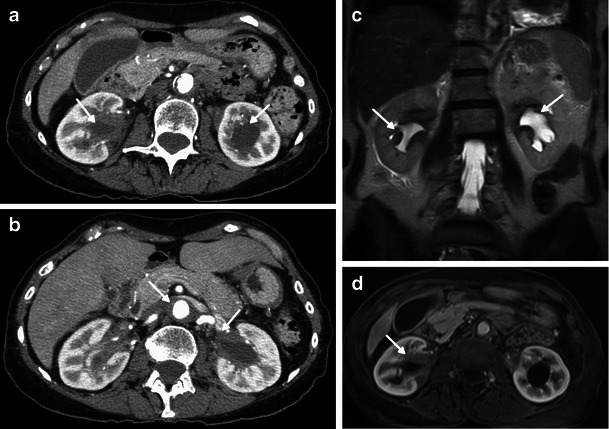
Axial enhanced CT images on a lower (a) and higher (b) abdominal level and coronal HASTE (c) and axial T1 post-gadolinium (d) MRI images in a 71-year-old female showing bilateral and symmetric infiltration of the renal sinuses, with mild hydronephrosis (arrows). Note also the periaortic concentric soft tissue infiltration (arrow)
